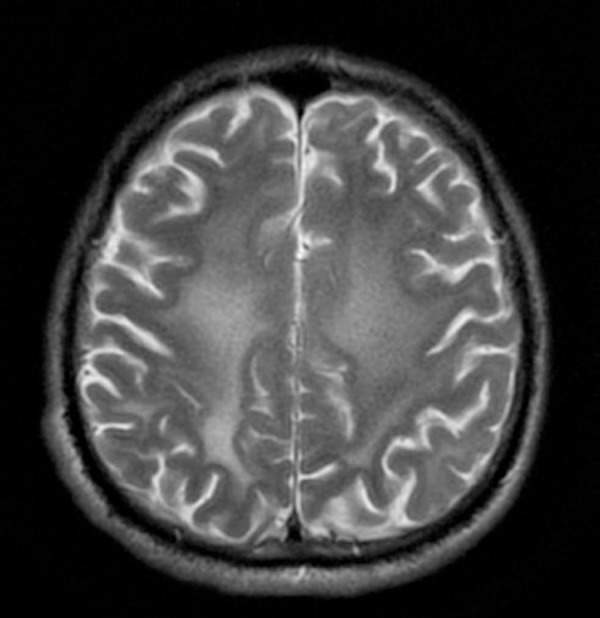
MRI T2W image of patient with widespread leukoencephalopathy involving
